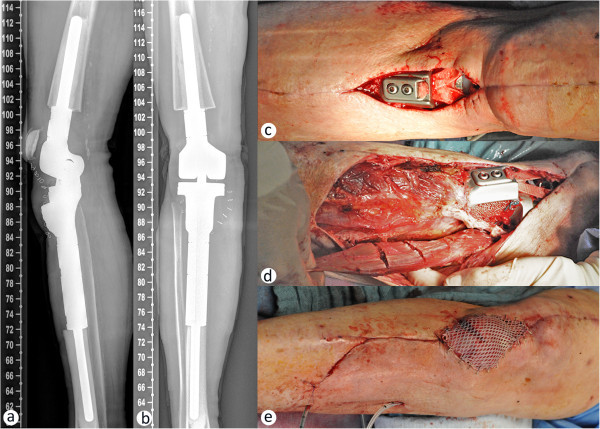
Soft tissue coverage following megaendoprosthetic knee reconstruction. Postoperative lateral (a) and anterioposterior (b) radiographs showing correct implant positioning. Due to the necessity of the patellar-ligament resection, absent soft tissue cover of the proximal anterior portion of the tibial implant caused wound-healing deficiencies, which required operative revisions (c). To achieve soft tissue coverage a medial gastrocnemius muscle flap was prepared (d) and covered with a mesh graft from the opposite thigh (e).
